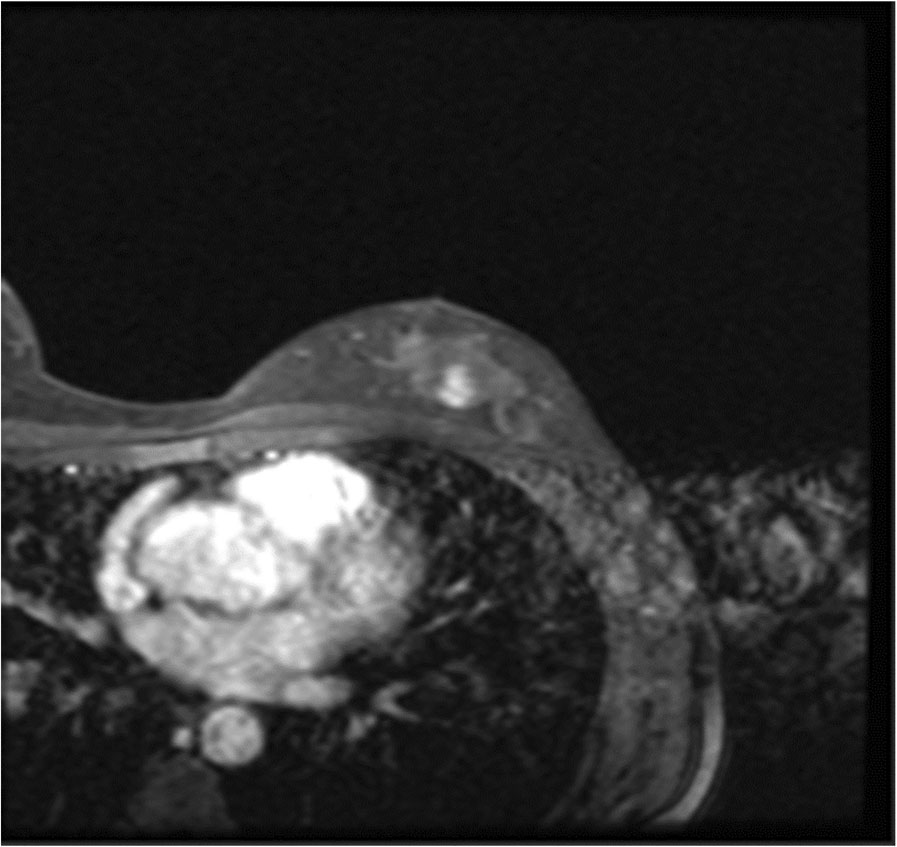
Contrast-enhanced magnetic resonance imaging (CE-MRI). CE-MRI showing an irregular mass with early arterial enhancement (14 mm × 11 mm × 12 mm) that was confined to the same area in the left C area
